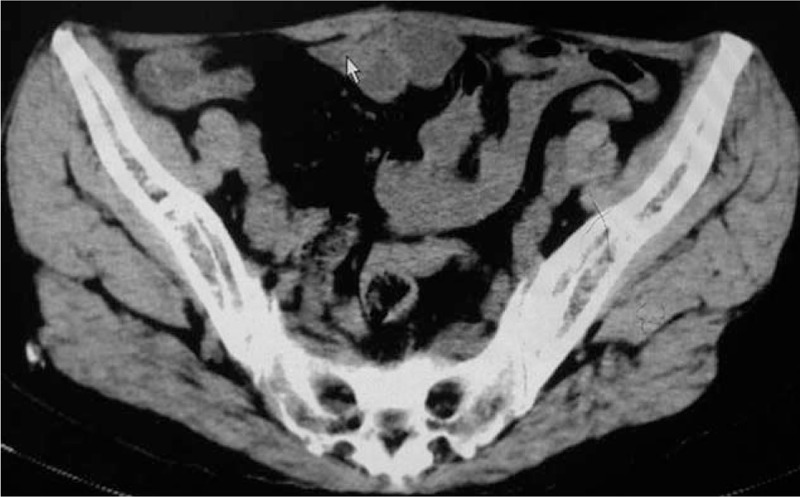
Abdominal CT showed no obvious expansion of the bowel. CT = computed tomography.
